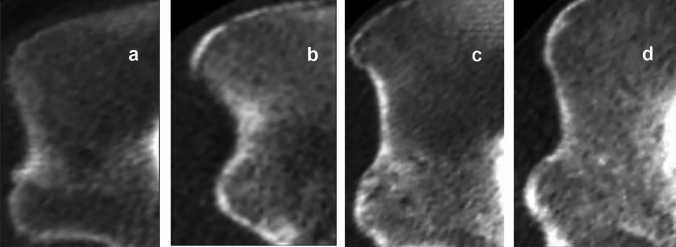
Computed tomography images depicting the stages of ossification and fusion of the iliac crest apophysis based on Kreitner’s four-stage classification [8]. a Stage 1—no ossification of the iliac crest apophysis. b Stage 2—iliac crest apophyseal ossification without any fusion with the ilium. c Stage 3—partial fusion. d Stage 4—complete fusion; image source: in-house archives
